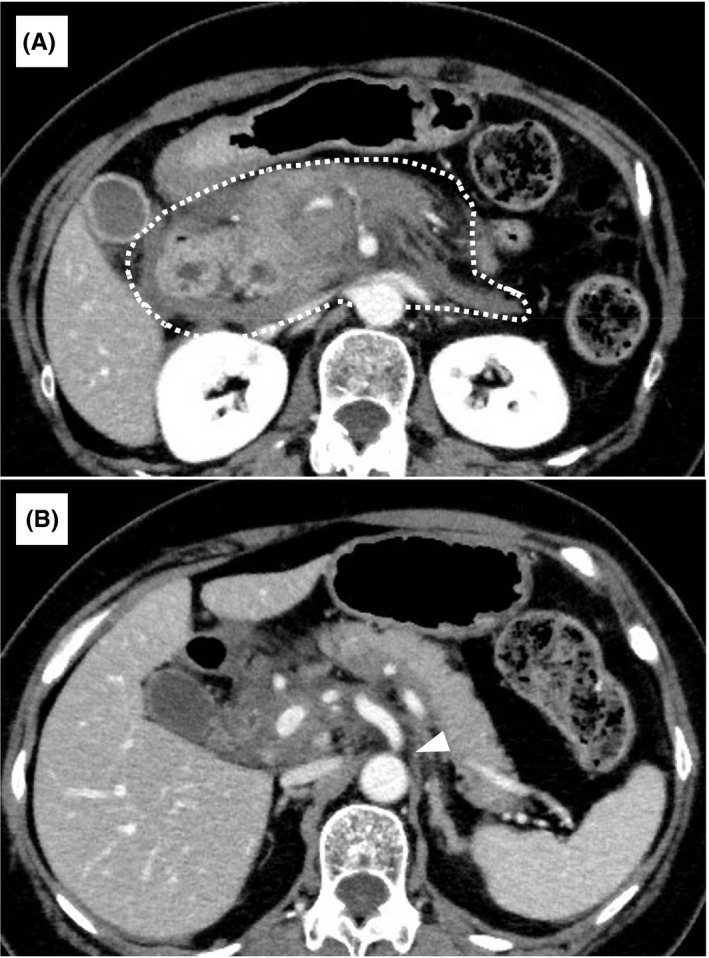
Contrast‐enhanced computed tomography of the abdomen on admission. A, A massive retroperitoneal hematoma below the pancreas and surrounding the pancreas (white dotted line). B, Celiac artery narrowing (white arrowhead)
